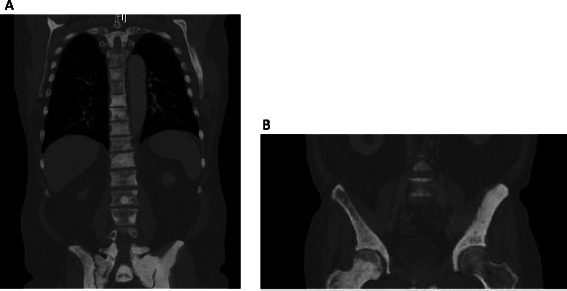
Diffuse sclerosis, resulting from the patient’s high osseous tumor burden and associated high radium-223 uptake. Diffuse osseous sclerosis on CT imaging of the spine (A), bilateral ilia (B), and bilateral femoral heads (B) is shown. This sclerosis occurred because of the patient’s high volume of bony metastatic disease and consequential high radium-223 uptake. We believe that this combination of high osseous tumor burden and high radium-223 uptake, resulting in diffuse sclerosis, contributed to our patient’s transfusion dependence, which has persisted in the absence of continued radium-223 therapy or systemic disease progression.
